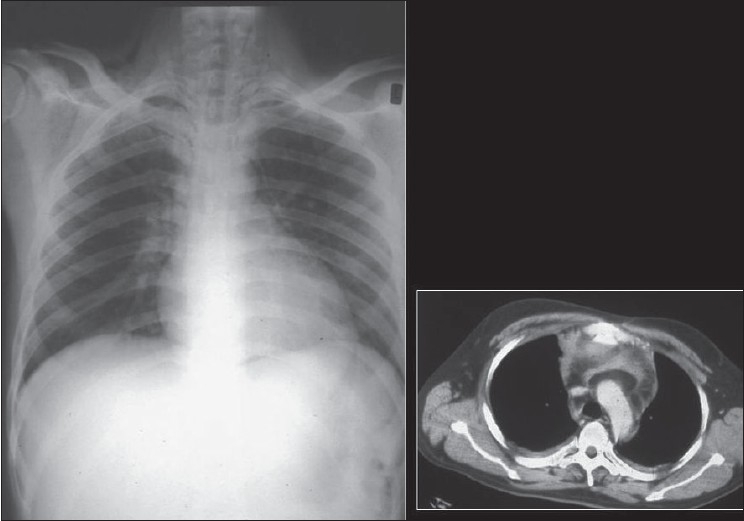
A frontal radiograph and axial CT depicting a pneumopericardium. Note the surgical emphysema at the root of the neck
